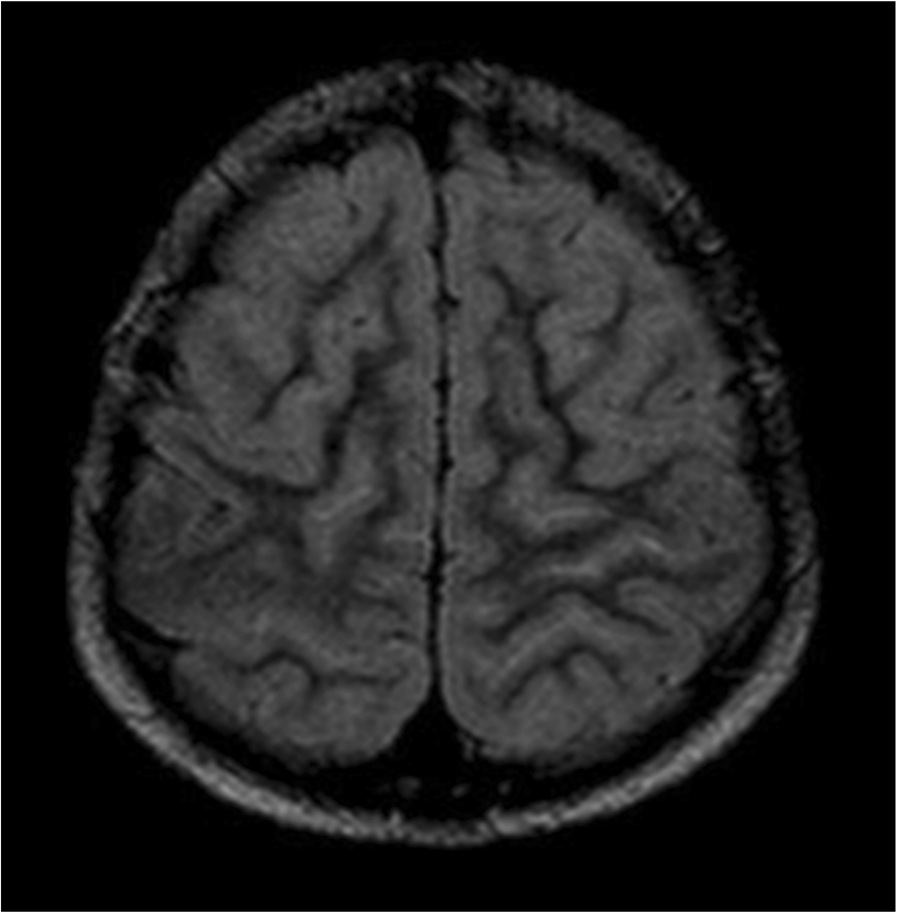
Axial FLAIR image in a 12-year-old girl with Down syndrome and moyamoya pattern depicting the classical *ivy sign*, corresponding to areas of absence of normal FLAIR suppression of CSF within cortical sulci. This sign probably represents slow flow in leptomeningeal collaterals. The exam was performed without general anesthesia
